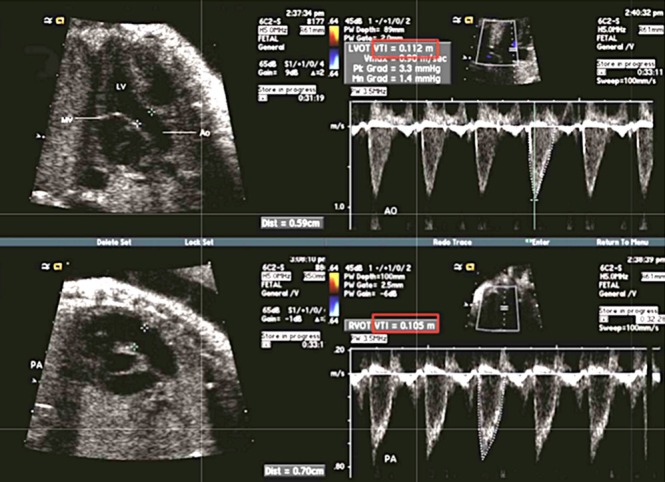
Calculation of fetal left and right ventricular outputs. Still‐frame 2D ultrasound images and Doppler spectra demonstrating the Doppler‐based method of calculating fetal ventricular stroke volumes and outputs. Aortic (top left) and pulmonary (bottom left) valve annuli are measured in systole in as close to an axial plane as possible. The cross sectional area (CSA, in cm) of the valve is then calculated using the equation π × (valve diameter/2)^2^. Pulsed‐wave Doppler interrogation through the aortic (top right) and pulmonary (bottom right) valves with the cursor aligned parallel to flow (and no angle correction) provides a spectral tracing for each valve, which are then planimetered to generate the velocity time integral (VTI) in cm. The ventricular stroke volume (SV) is calculated using CSA × VTI (in cm^3^ or mL) and ventricular output is calculated using SV × fetal heart rate. By combining the ventricular outputs (usually measured in units of mL/min and indexed by estimated fetal weight (mL/kg/min)), the combined cardiac output can be derived. Ao, aorta; LV, left ventricle; LVOT, left ventricular outflow tract; MV, mitral valve; PA, pulmonary artery; RVOT, right ventricular outflow tract.
